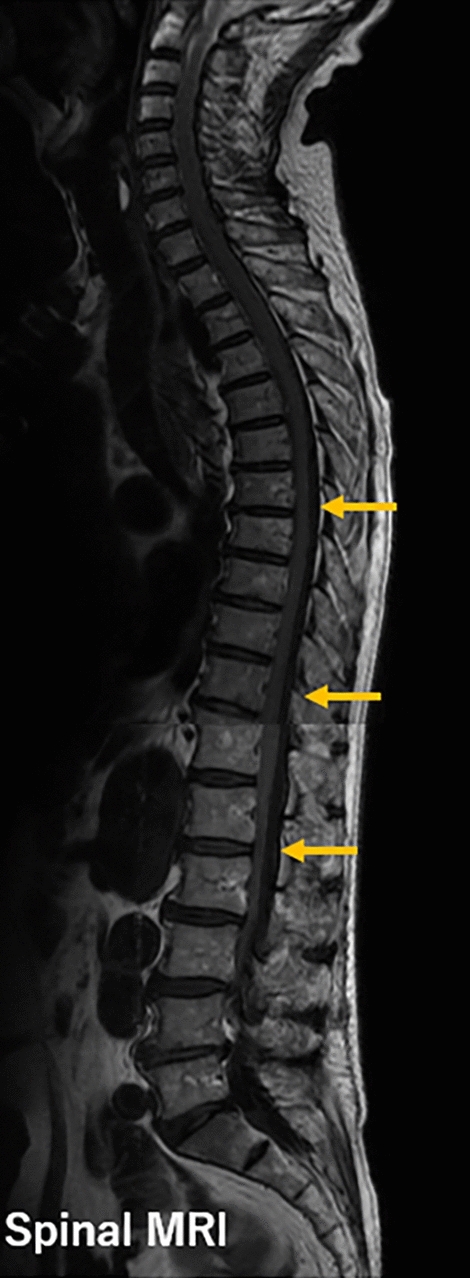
Magnetic resonance imaging of the spinal subdural hematoma. The arrow indicates the presence of spinal subdural hematoma
